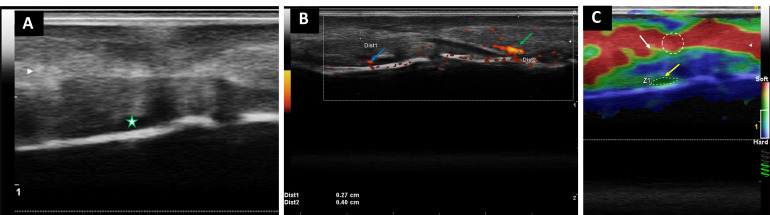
Multimodal ultrasound assessment of palmar plate enthesitis at the right third distal interphalangeal (DIP) joint. (A) B-mode ultrasound of the volar aspect of the right third DIP joint demonstrates hypoechoic thickening at the insertional enthesis of the palmar plate with adjacent anechoic effusion (star), consistent with ultrasound-defined enthesitis according to OMERACT criteria. (B) Power Doppler acquisition was performed with the same technical parameters as those described in Figure 2. Power Doppler imaging reveals a vascular signal at the insertional enthesis of the palmar plate (blue arrow) and at the deep digital flexor tendon insertion (green arrow), supporting active enthesitis and flexor tendon–related inflammatory involvement. (C) Strain elastography demonstrates heterogeneous stiffness distribution at the insertional enthesis and adjacent perientheseal tissue. Elastography was acquired using light repetitive manual compression with real-time quality control monitored by the calibrated green spiral indicator. Three consecutive compression cycles were performed, and semiquantitative measurements were obtained from regions of interest (ROIs; 0.02–0.03 cm^2^) positioned over the insertional enthesis and adjacent reference tissue for internal comparison at comparable depths within the elastographic field to ensure consistent semiquantitative comparison, with ROI placement guided by the corresponding B-mode anatomical reference and avoiding inclusion of fluid components or acoustic artifacts. The insertional enthesis exhibited hard component values ranging from 79.9% (white arrow, perientheseal extension) to 100% (yellow arrow, insertional enthesis) (%HRD), whereas adjacent structurally normal reference tissue demonstrated markedly lower stiffness (4.5% HRD). Strain ratios ranged from 0.42 to 2.15, indicating biomechanical heterogeneity consistent with active inflammatory enthesitis. These findings are consistent with ultrasound-defined digital enthesitis and flexor tendon–related inflammatory involvement, overlapping with domains incorporated into the GLobal OMERACT Ultrasound DActylitis Score (GLOUDAS) framework [9]. Articular inflammatory manifestations have also been described in association with HS [10].
